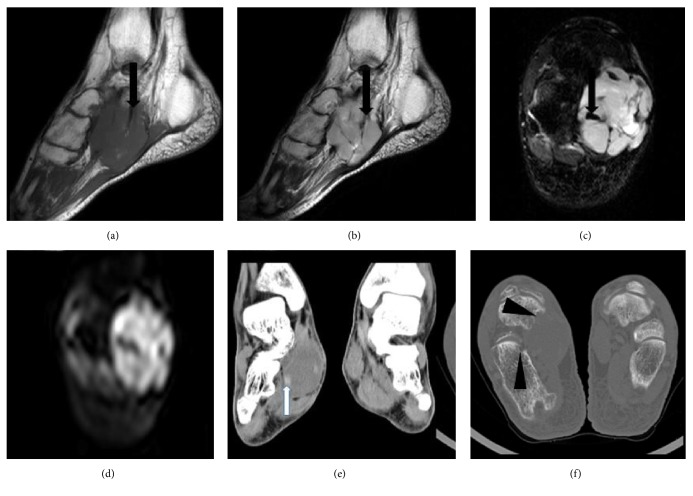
A 46-year-old female with a poorly differentiated adult fibrosarcoma*. (a, b) Sagittal T1WI and T2WI exhibited a lobulated long T1 and mixed T2 signal mass in the right plantar deep fascia, with band-like areas of long T1 and short T2 signal (black arrows in (a) and (b)) indicating tumor fibrous septa. (c, d) Axial fat suppressed FSE T2WI and DWI show mixed signals, with more nodular short T2 signals (black arrow in (c)). The high lesion signal on DWI indicated dense cellularity. (e, f) Reformatted coronal images of soft tissue and axial bone images displaying an iso-attenuating soft tissue mass, with a slightly higher density small nodule (white arrow in (e)) and partial bone destruction (arrow heads in (f)).
